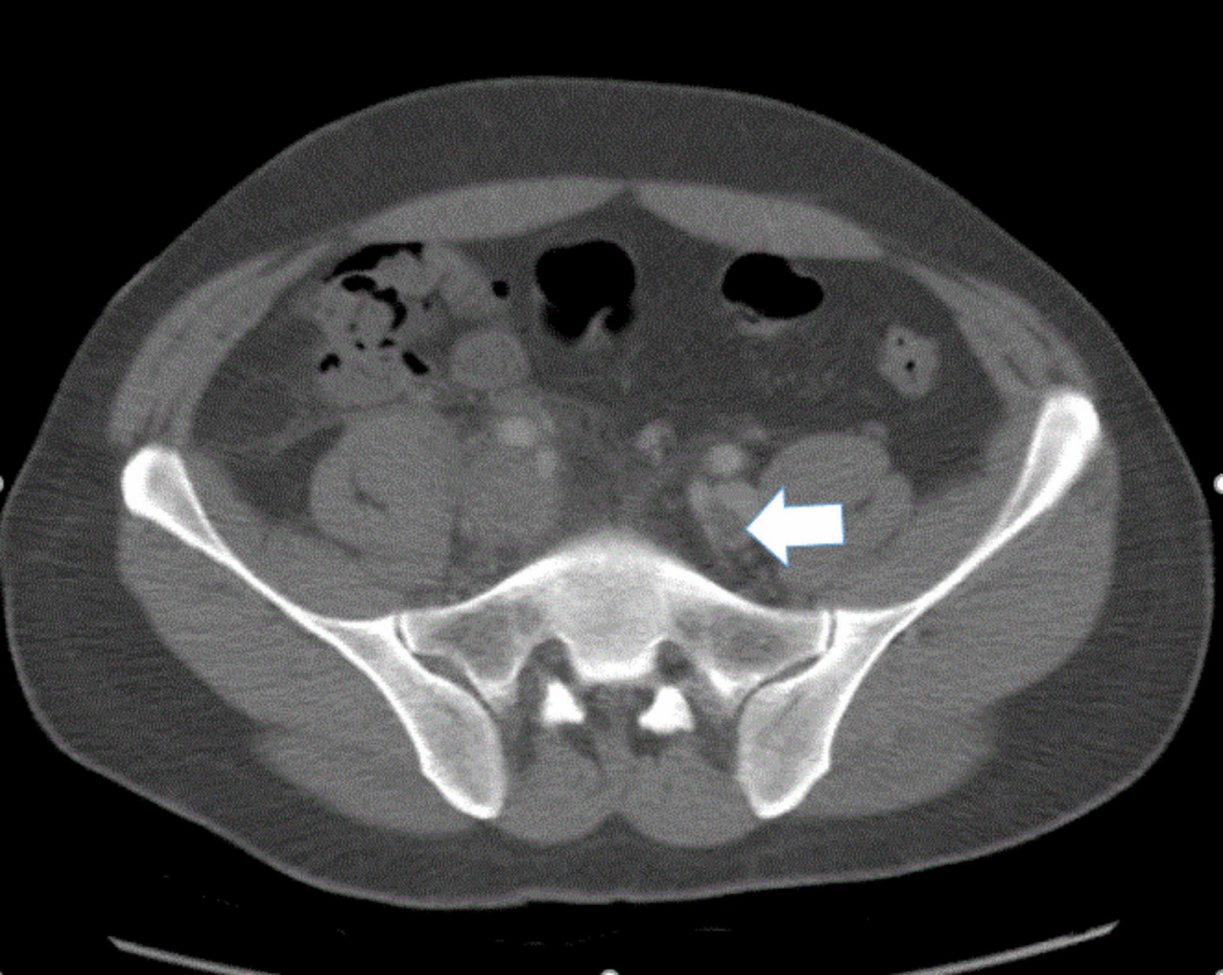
Computed tomography of the abdomen and pelvis with IV contrast, in an axial view, shows left external iliac vein thrombosis (white arrow)
